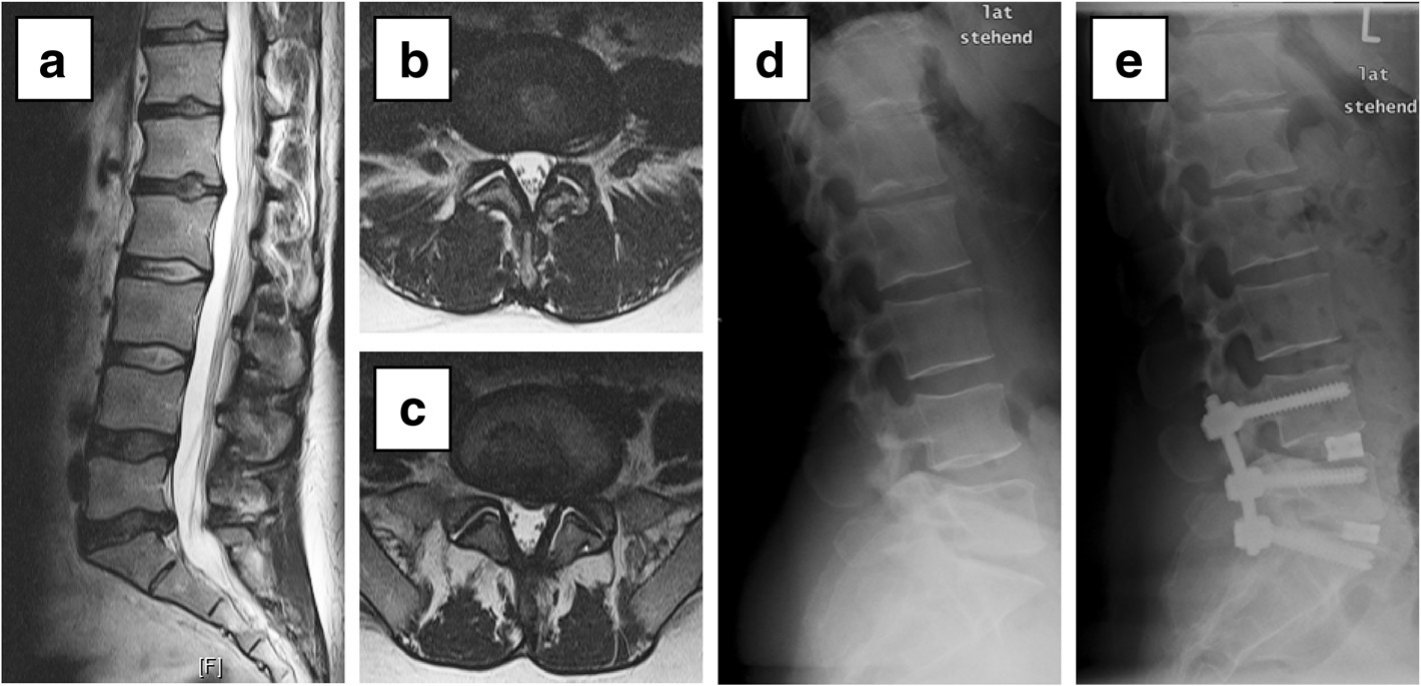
Extension of surgical scope by one motion segment cranially. a-c T2-weighted magnetic resonance images preoperatively: a lumbar sagittal image and b axial lumbar image of L4/5 and c L5/S1. d Lateral radiographic image preoperatively and e postoperatively. The patient presented with chronic lumbar back pain and sciatic pain on the left side and a sensorimotor deficit of 4/5 on the Medical Research Council scale for muscle power for ankle dorsiflexion, plantarflexion, and toe extension. Advanced intervertebral disc degeneration was observed between L5 and S1 (Pfirrmann grade 4) and a median intervertebral disc protrusion at L5/S1. Mild facet joint degeneration was seen at L4/5 (Weishaupt grade 0 right and grade 1 left facet) and almost no facet joint degeneration at L5/S1 (Weishaupt grade 0 bilaterally). Recommendation before inpatient gradual diagnostics (IGD): Lumbar fusion at L5/S1 with transforaminal lumbar interbody fusion (TLIF). Results obtained during IGD: • bilateral facet joint infiltration of L5/S1: 70 % reduction of back pain. • epidural injection of L5/S1: 40 % improvement of sciatic pain. • bilateral facet joint infiltration of L4/5: 60 % reduction of back pain. Recommendation after IGD: lumbar fusion of L4-S1 with TLIF. At 1 year follow-up, the patient reported no pain
